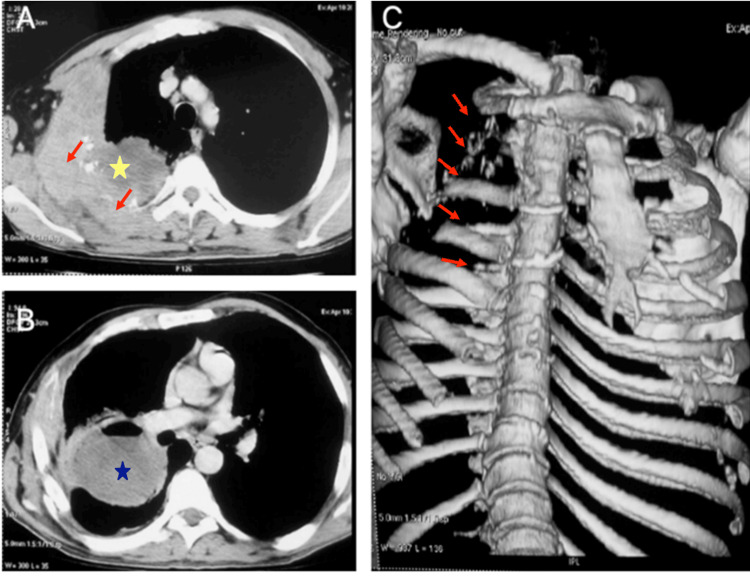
Chest computed tomography. Axial images in the mediastinal window (panels A and B). Panel A shows a peripheral right upper lobe mass (yellow asterisk) with direct chest wall invasion, involving adjacent soft tissues and the arcs of the first through fifth ribs (red arrows). Panel B demonstrates central cavitation within the mass (blue asterisk), suggestive of tumor necrosis or superimposed infection. A three-dimensional bone reconstruction (panel C) depicts the lytic destruction of the right first through fifth ribs (red arrows). Tumor stage at the time of imaging was T4N0Mx.
